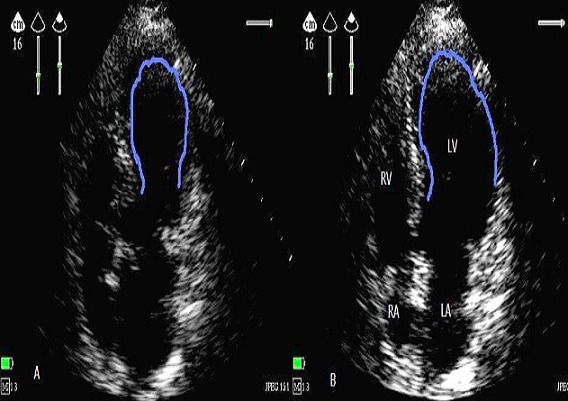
Echocardiogram on admission demonstrated apical akinesis with hyper kinesis basal and ballooning of the left ventricle apex (blue arc); A End-systolic phase, B End-diastolic phase.(LA: left atrium, LV: left ventricle, RV: right ventricle, RA: right atrium)
